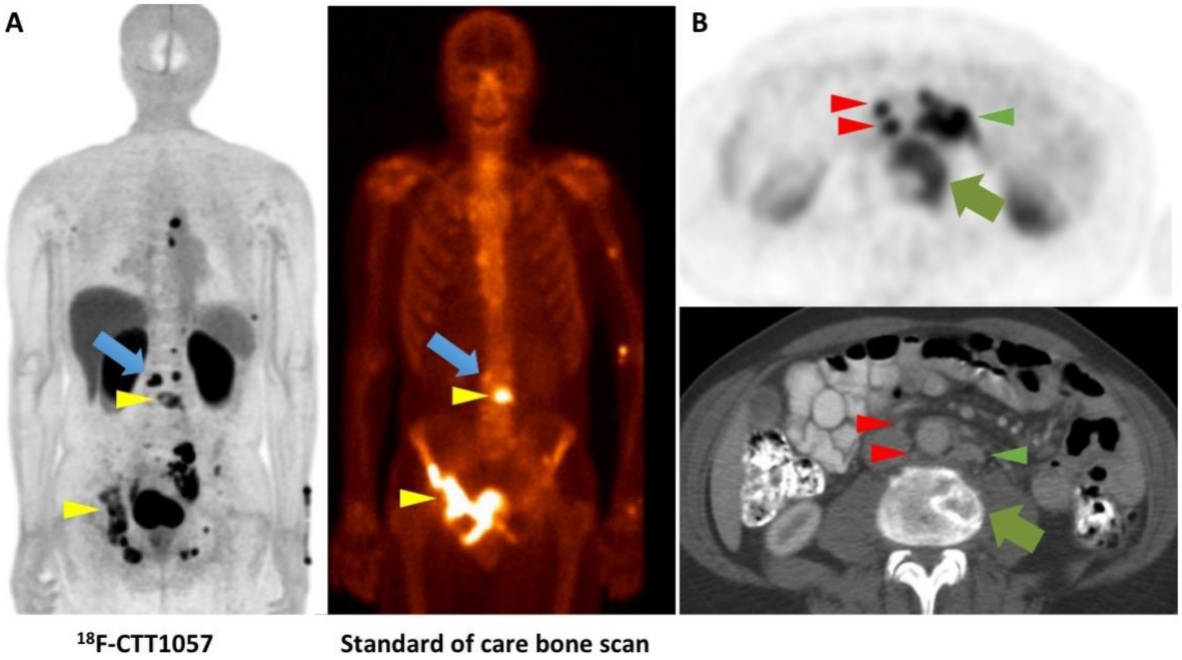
Head-to-head comparison of [^18^F]CTT1057 PET study vs. standard of care bone scan vs. conventional CT. (A) [^18^F]CTT1057 maximum intensity projection (MIP) PET (left) with several matching PSMA avid osseous lesions on standard of care bone scan (right, yellow arrowheads), which can be seen on both imaging modalities. However, a PSMA avid lesion on [^18^F]CTT1057 in the skeleton has no clear bone scan correlate (blue arrow). (B) Axial [^18^F]CTT1057 PET (upper row, red arrowheads) highlights a 3 mm lymph node that is not enlarged by size criteria on conventional CT (lower row), but has marked [^18^F]CTT1057 uptake. In addition, further enlarged PSMA avid retroperitoneal lymph nodes can be detected (red arrowheads), along with PSMA avid lytic osseous metastases (green arrows).
